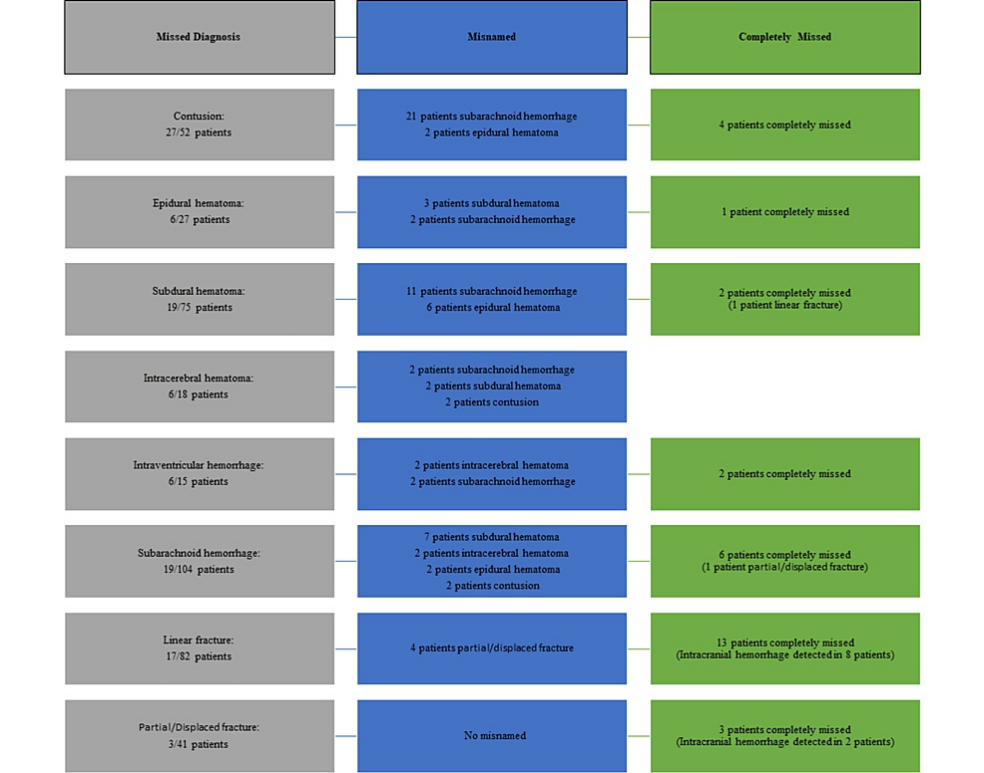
Assessment of discordant diagnoses in brain CT imaging.
